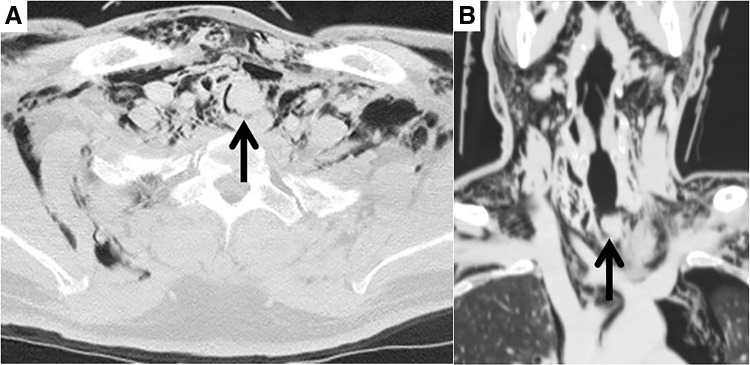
Chest computed tomography scan showing severe subcutaneous and mediastinal emphysema and tracheal injury with a 13-mm endotracheal mass (arrow). (A) Horizontal section. (B) Coronal section.
